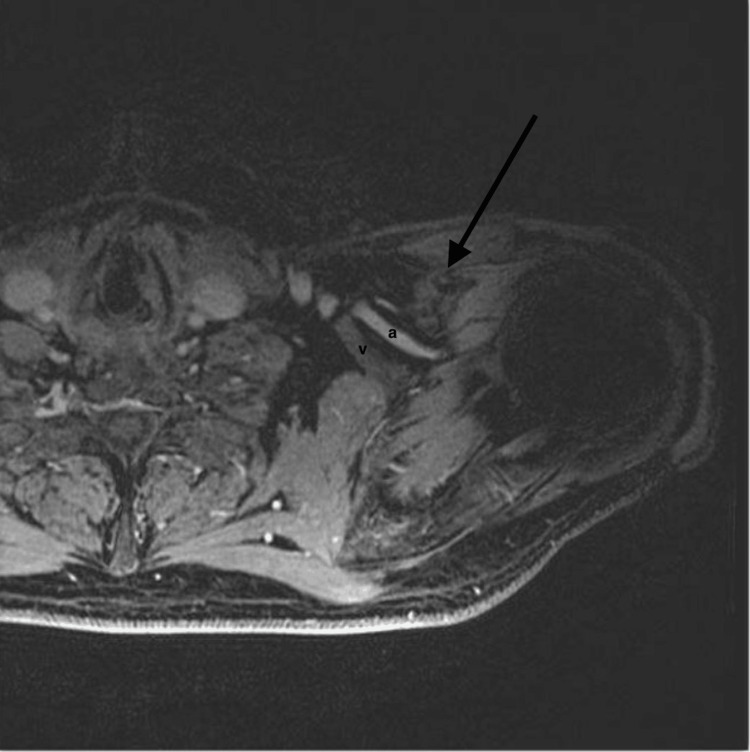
MRI The black arrow points to the left axillary lipomatous mass, showing mass effect on the nearby axillary vasculature (a, v). a, artery; v, vein
